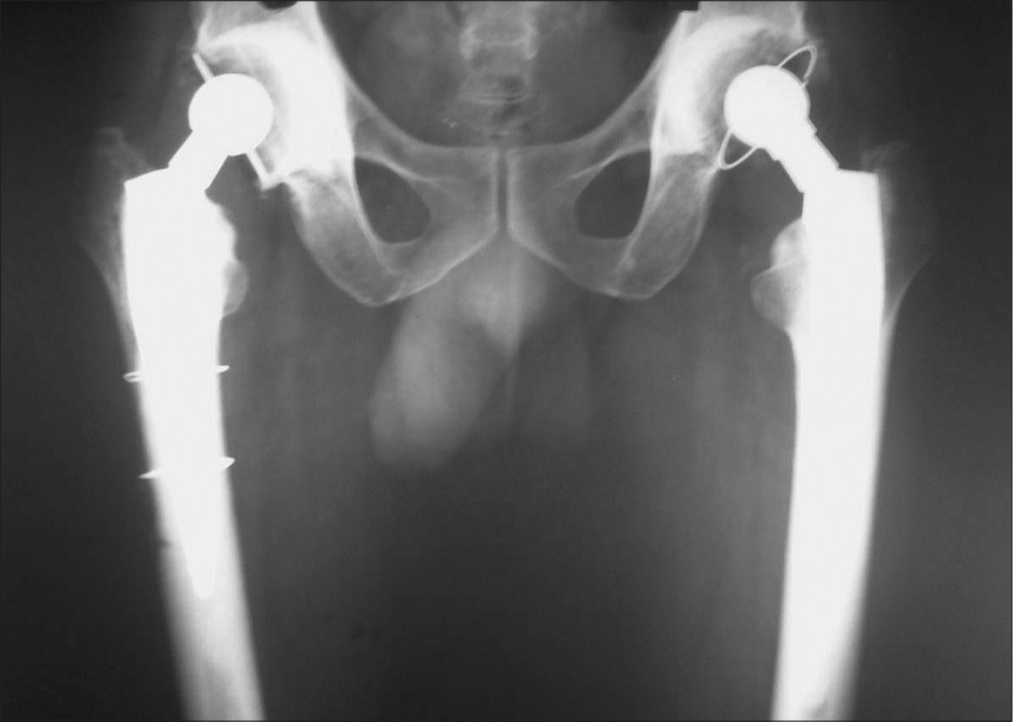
X-ray pelvis with both hips of the same patient, 6 months after the Muller cemented total hip arthroplasty of right hip. The patient sustained intra-operative periprosthetic fracture which was treated with encirclage
